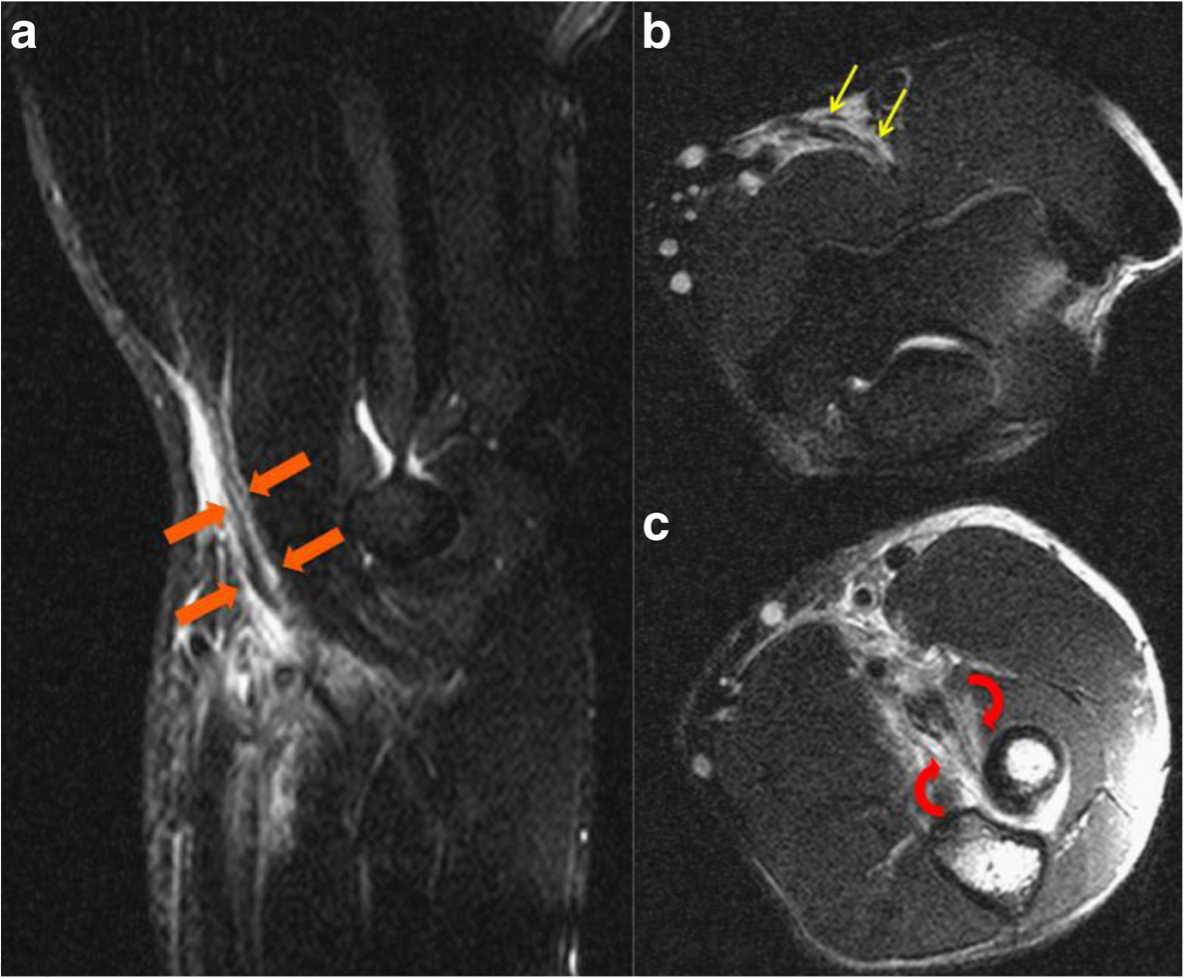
Magnetic resonance imaging of the left elbow. (a) Sagittal short tau inversion recovery image reveals high signal edema surrounding an attenuated distal biceps brachii tendon (thick orange arrows). (b, c) Axial fat-suppressed fast spin echo T2-weighted images demonstrate some linear high-signal intensity within the attenuated distal biceps brachii tendon consistent with longitudinal partial-thickness tearing (thin yellow arrows in b). At the level of the radial tuberosity, there is a full thickness tear at the tendon insertion (curved red arrows in c) with 1cm to 2cm of tendon retraction.
